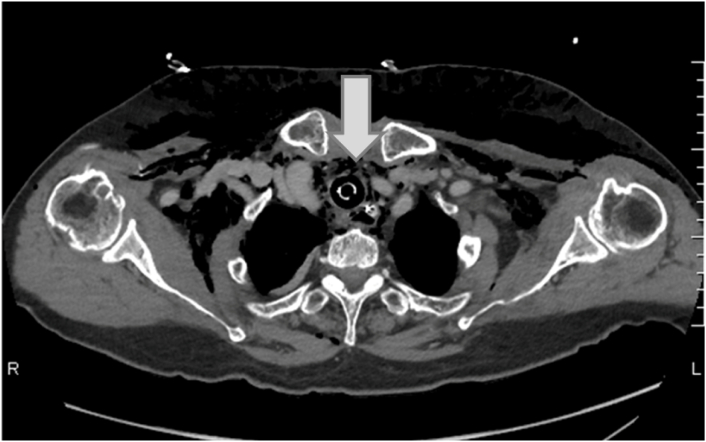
Enhanced computed tomography performed on admission showing a partial wall irregularity or a defect (arrow) in the anterior wall of the trachea.
